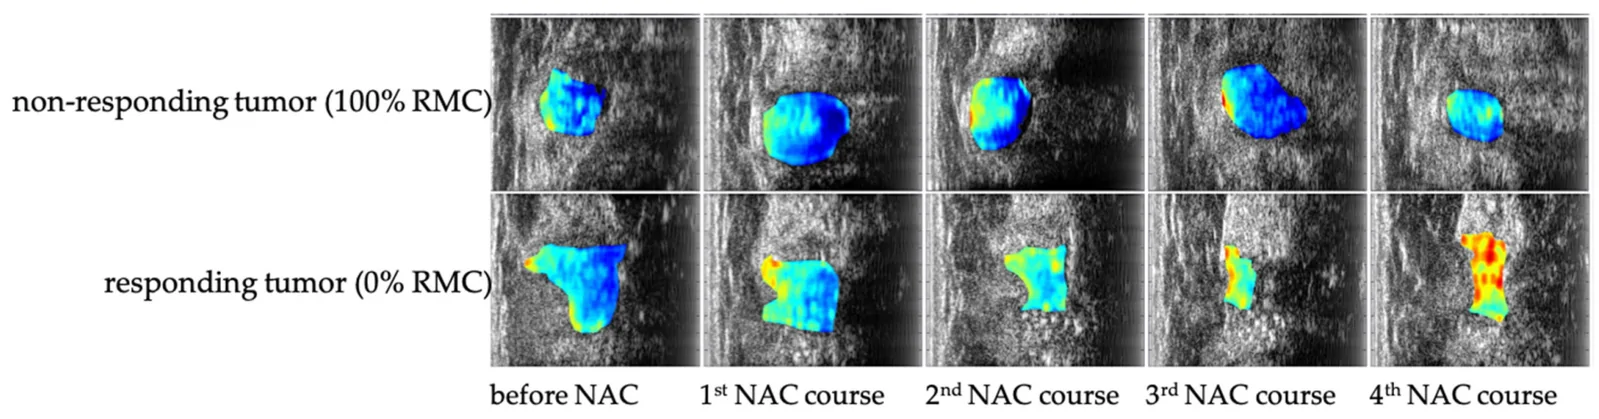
Representative quantitative ultrasound (QUS) parametric maps based on the integrated backscatter coefficient (IBSC) overlaid on B-mode images of breast tumors during neoadjuvant chemotherapy (NAC). The upper row shows IBSC parametric maps from a patient exhibiting no response to NAC, characterized by persistently low and spatially homogeneous backscatter values.
